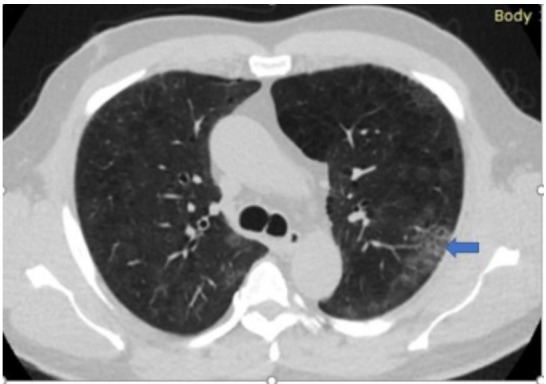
An axial non-enhanced chest CT scan of a 62-year-old male with fever and cough of 3-days duration. Lung window image shows “crazy paving pattern” (interlobular & intralobular septal thickening) in the periphery of the left lower lobe (arrow) and subtle patchy bilateral ground-glass opacities. Was found to be RT-PCR positive for COVID-19.
